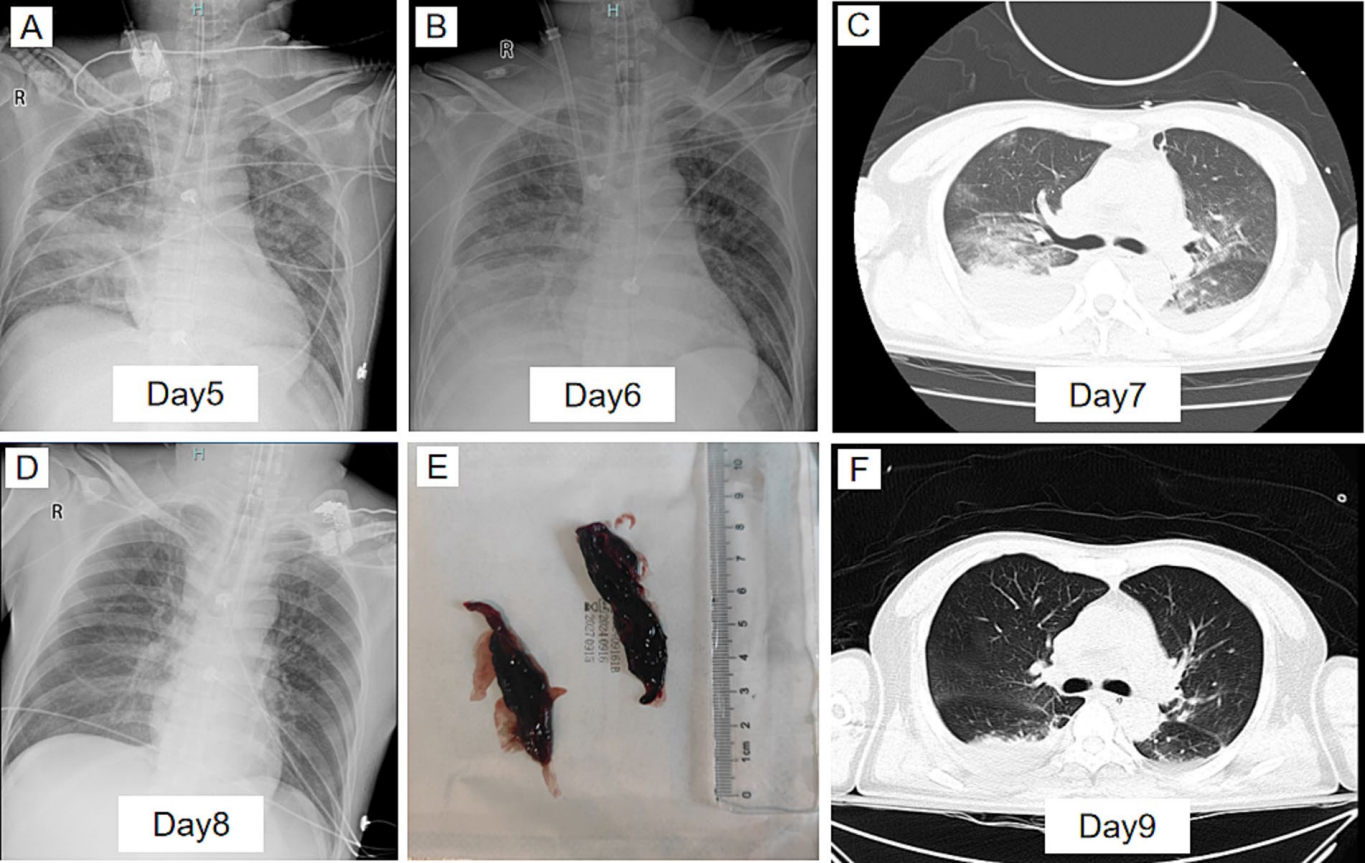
Dynamic imaging during and after VV-ECMO treatment. (A) Chest X-ray on day 5 showing progressive lung consolidation and bilateral infiltrates, indicating ongoing pulmonary infection. (B) Chest X-ray on day 6 showing persistent bilateral lung infiltrates with worsening pulmonary involvement. (C) Chest CT scan on day 7 showing significant consolidation in both lungs, indicating the progression of the pulmonary infection. (D) Chest X-ray on day 8 showing improvement in lung consolidation, with a reduction in pulmonary infiltrates. (E) Fiberoptic bronchoscopy showing blood clots aspirated from the airways. (F) Chest CT scan on day 9 after ECMO weaning showing significant improvement in lung conditions with reduced consolidation and improved airspaces.
